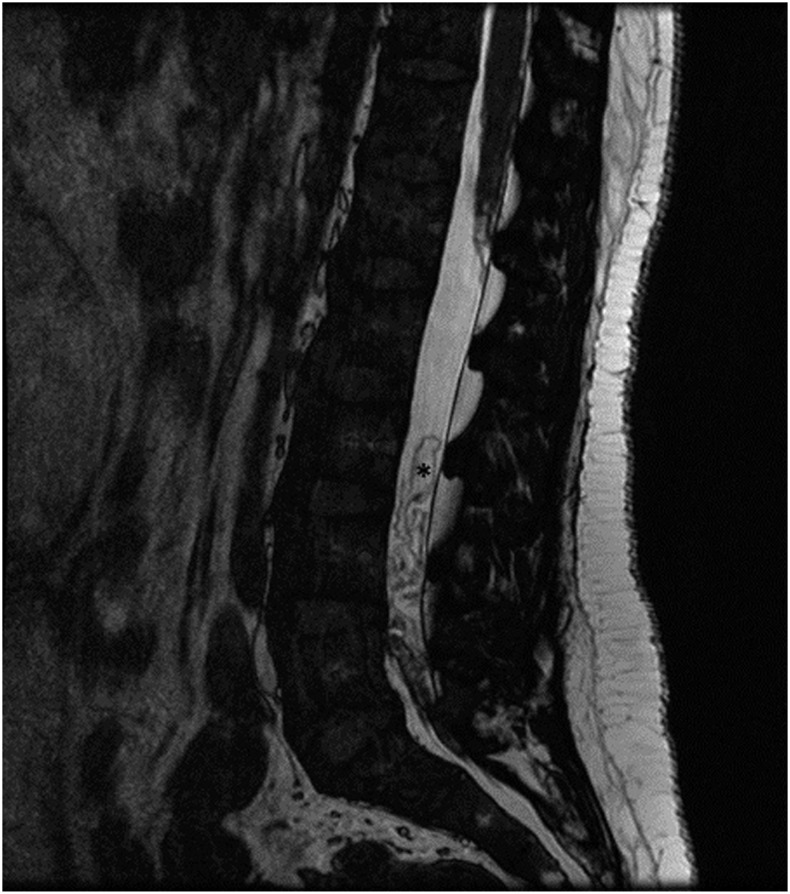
Spinal MRI after antiparasitic treatment; volumetric T2-weighted imaging in the sagittal plane shows clustered membranes resembling the folding of cysts (asterisk).
